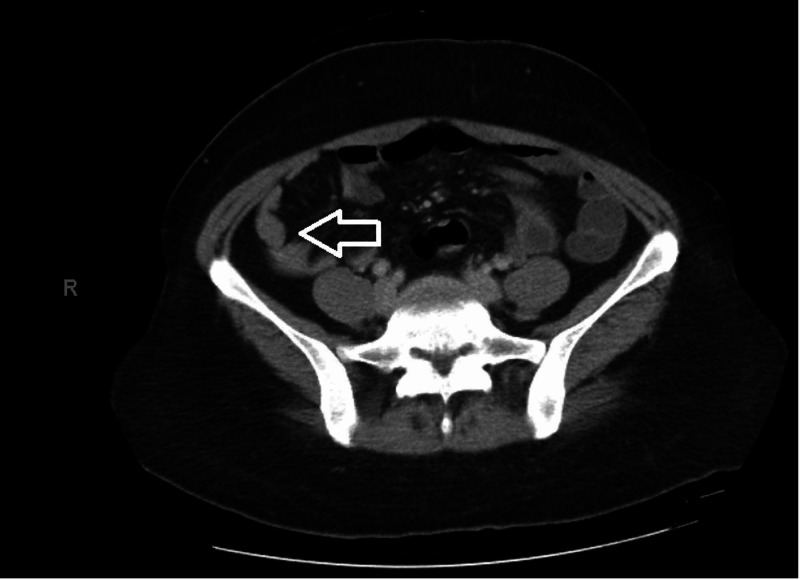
CT abdomen and pelvis showing right colonic wall patchy thickening consistent with colitis
